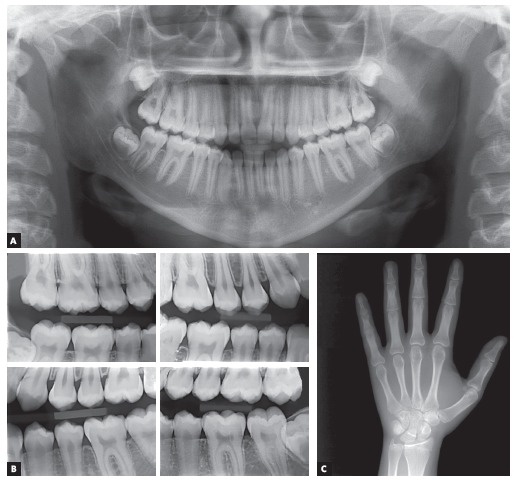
Baseline panoramic (A), interproximal (B) and hand and wrist (C) radiographs.
